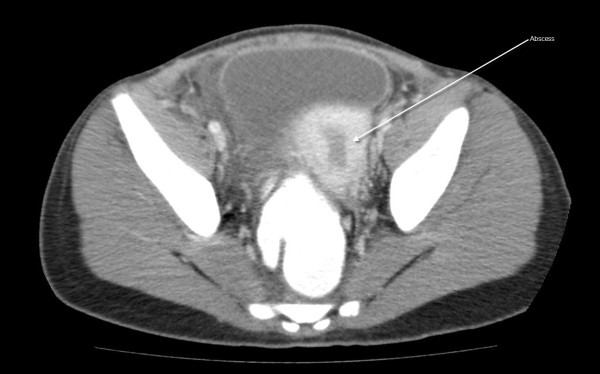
CT scan of the abdomen and pelvis with intravenous and oral contrast. Abscess is shown.
